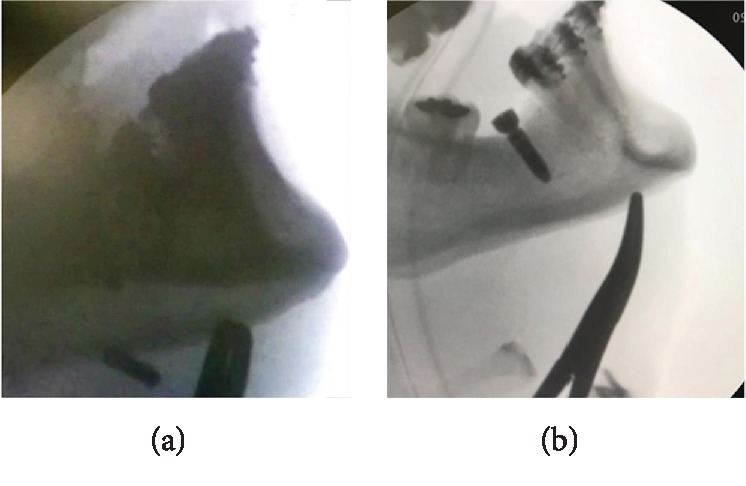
(a) X-ray profile showing location of the dental implant in the submandibular region. (b) X-ray showing clamping and removal of the dental implant.
